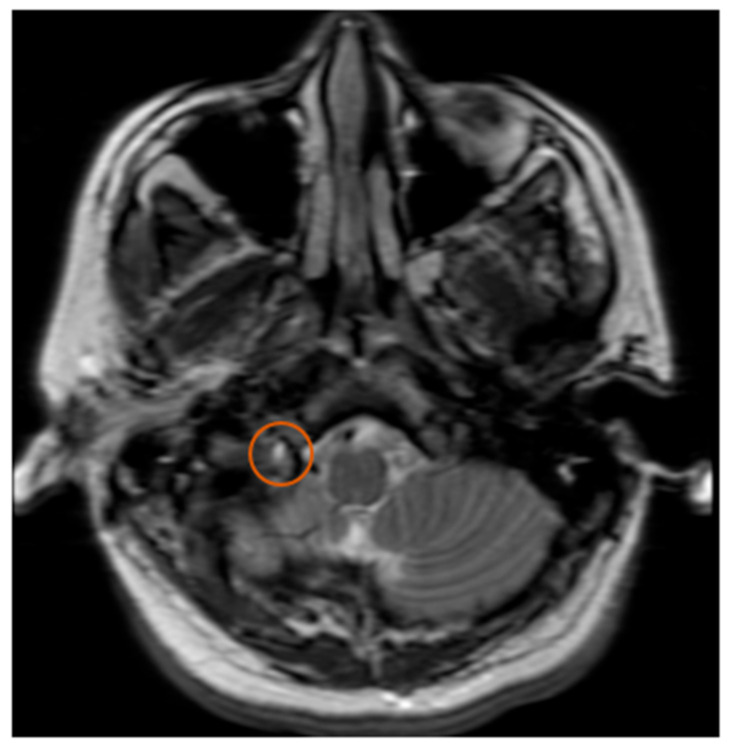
MRI T2. Orange circle indicates edema at the junction of the Jugular Foramen and the Hypoglossal Canal.
